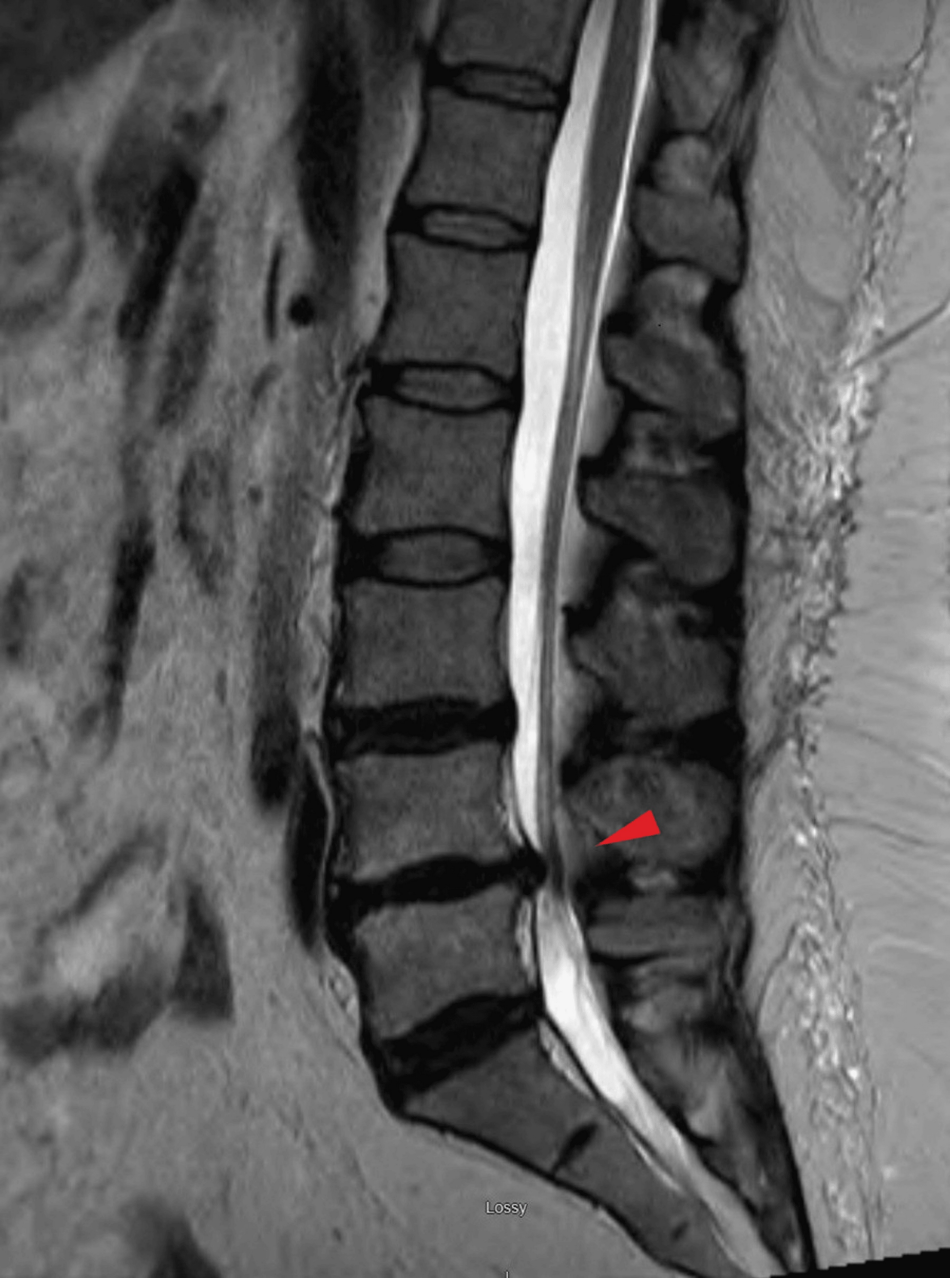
MRI of the lumbar spine shows L4-5 degenerative disc disease and diffuse annular bulging: degenerative facet, flaval ligament hypertrophy, severe central canal compromise (Age: 49 years).
